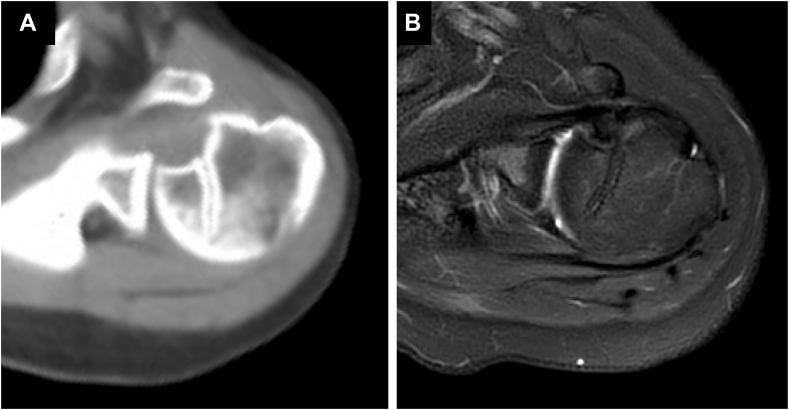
(A) Postoperative CT and (B) T2-weighted fat-suppressed MRI images of Case 1. CT scans were obtained 3 months after surgery, and MRI scans were acquired 6 months after surgery, confirming maintained reduction and successful attachment of the subscapularis to the reverse Hill-Sachs lesion. *CT*, computed tomography; *MRI*, magnetic resonance imaging.
